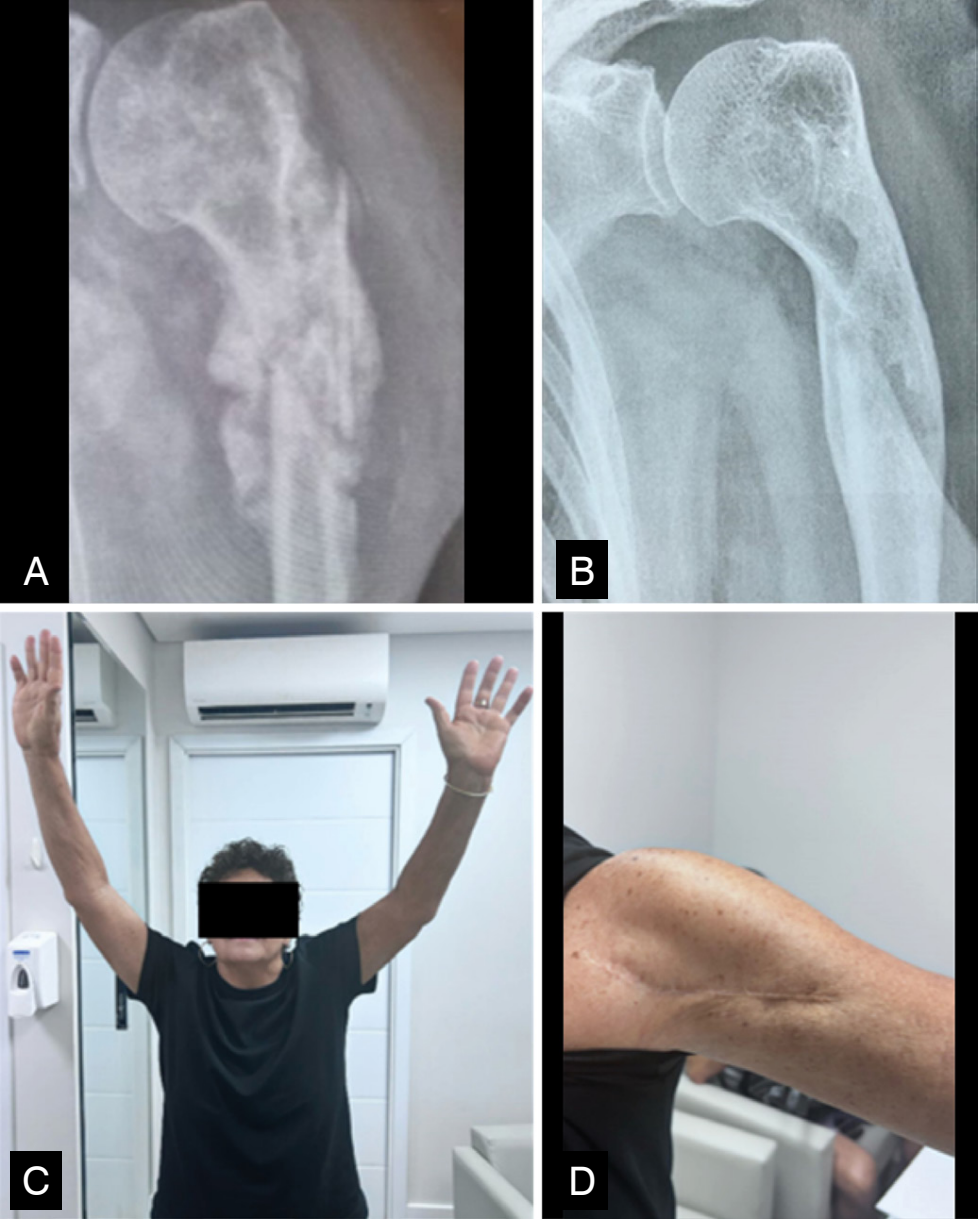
X-rays of patient 11 showing infected hypertrophic pseudoarthrosis (A) and consolidation (B) after treatment of infection; and clinical images of functionality (C) and scar (D).
